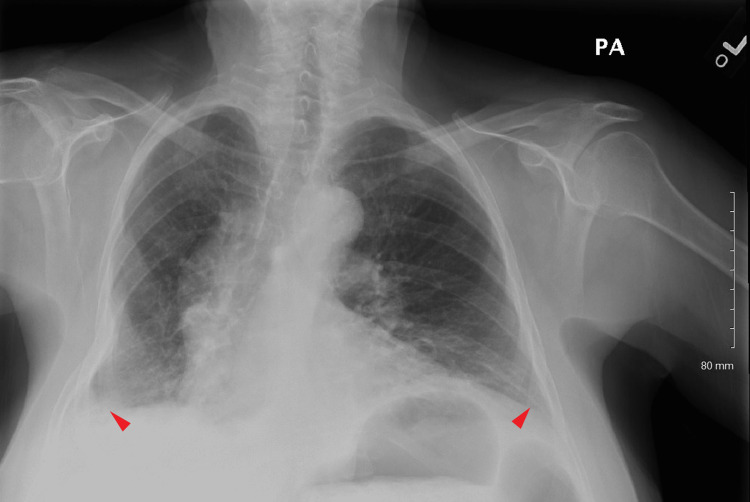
Chest X-ray showing small bilateral pleural effusions two days after discharge, 19 days post LNF LNF: laparoscopic Nissen fundoplication
